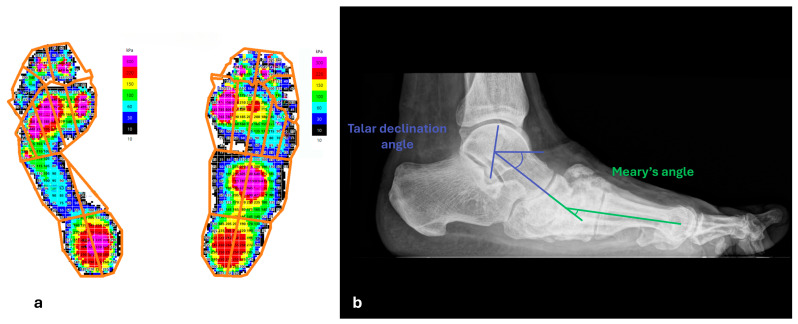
Plantar pressure regional masks of left foot and right foot (a) and representative radiographic measurements from a weight-bearing right foot radiograph (b) used in the present study, from a single participant at diagnosis. (a) Averaged maximum barefoot plantar pressure maps of both feet showing the 12 regional masks. The medial midfoot and lateral midfoot regions are indicated as the primary regions of interest on the right foot. (b) Weight-bearing lateral radiograph of the right foot with the measurements of the talar declination angle and Meary’s angle annotated. Additional radiographic measurements analyzed in the study (calcaneal pitch, cuboid height, and calcaneal-fifth metatarsal angle) are not shown for clarity.
